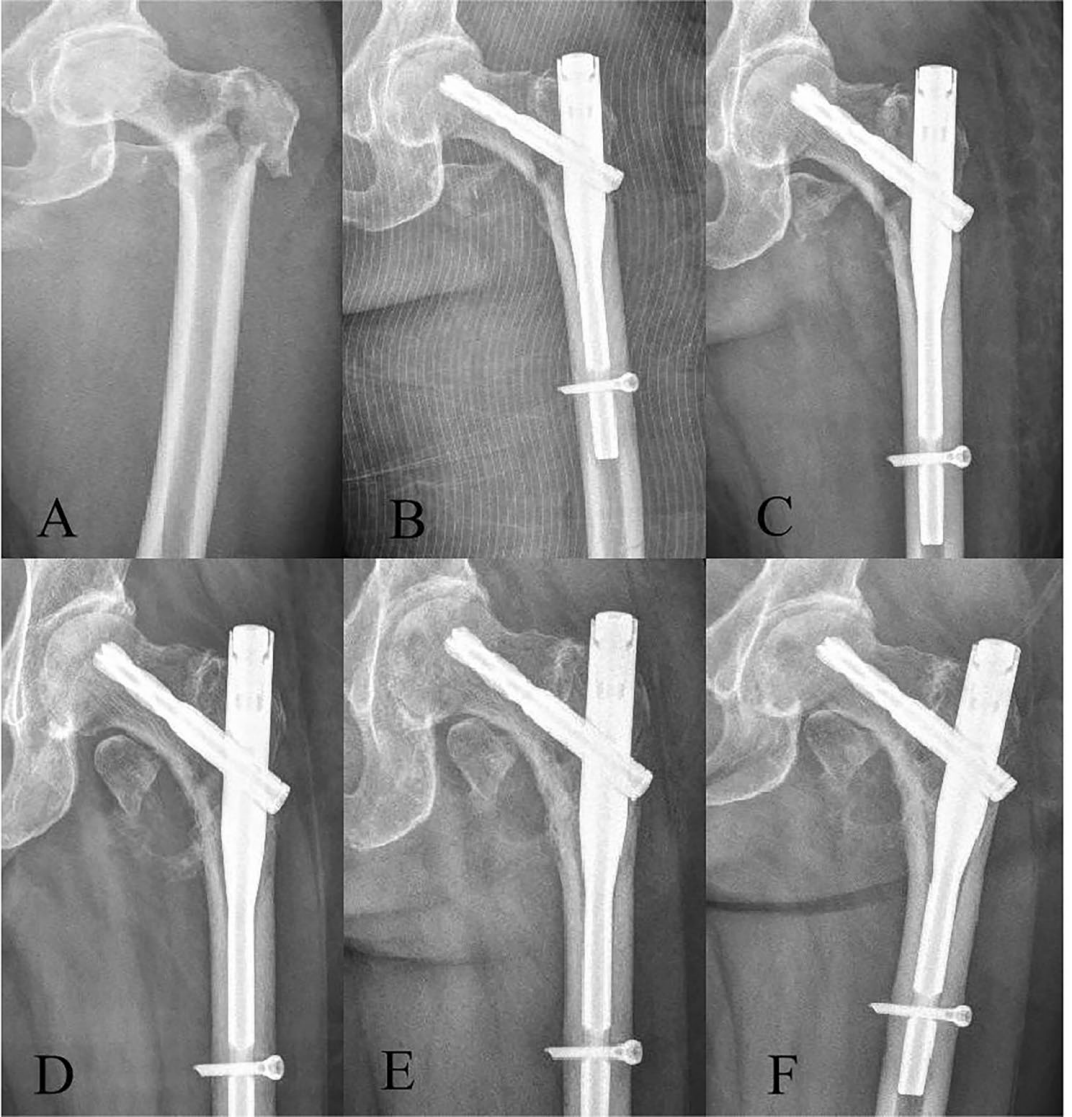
Dynamic observation of X-ray images of fractures. (A) Preoperative X-ray; (B) postoperative day 1 X-ray;(C) 1-month postoperative X-ray;(D) 3-month postoperative X-ray;(E) 6-month postoperative X-ray;(F) 12-month postoperative X-ray
